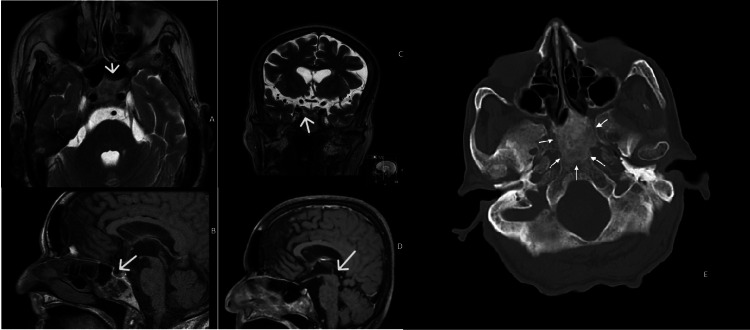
Axial (A) and coronal (B) T2-weighted images, and sagittal T1-weighted images before (C) and after (D) contrast administration. The lesion has a heterogeneous signal on spontaneous T1 (C) and T2 images (A and B) – it is slightly hyperintense on T2 and markedly hyperintense on T1 peripherally, and iso/hypointense on T2 and hypointense on T1 centrally. On postcontrast images, there is a diffuse enhancement (D). Axial bone window CT image showing an ill-defined ground-glass density lesion centered in the clivus, with loss of normal bone cortication; The lesion has a maximum antero-posterior extension of 35mm, but there is no significant mass effect. (1-E)
